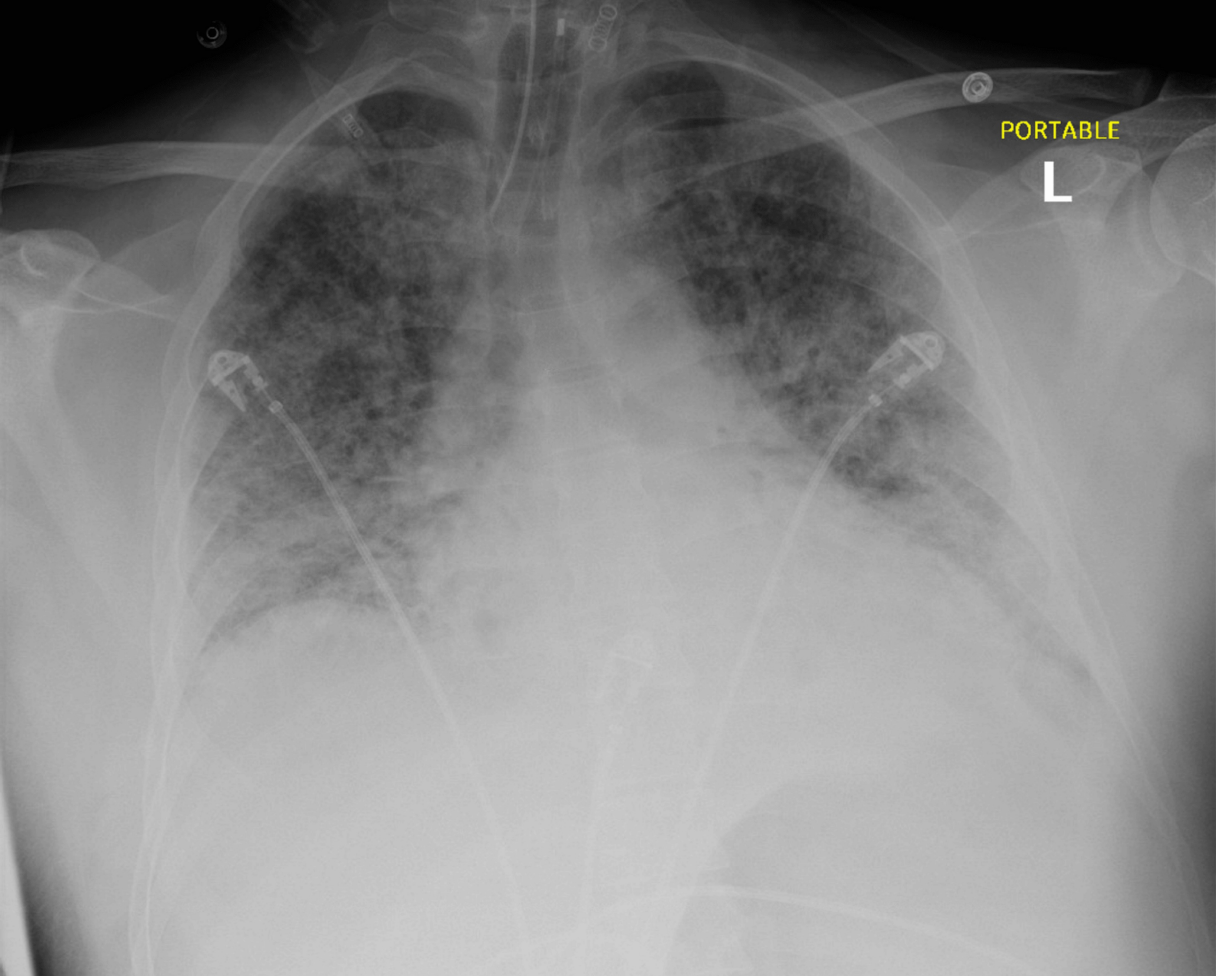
Chest X-ray showing interval intubation with the distal tip of the endotracheal tube projecting 4.4 cm above the carina Diffuse multifocal patchy airspace opacities represent pulmonary edema versus multifocal pneumonia.
